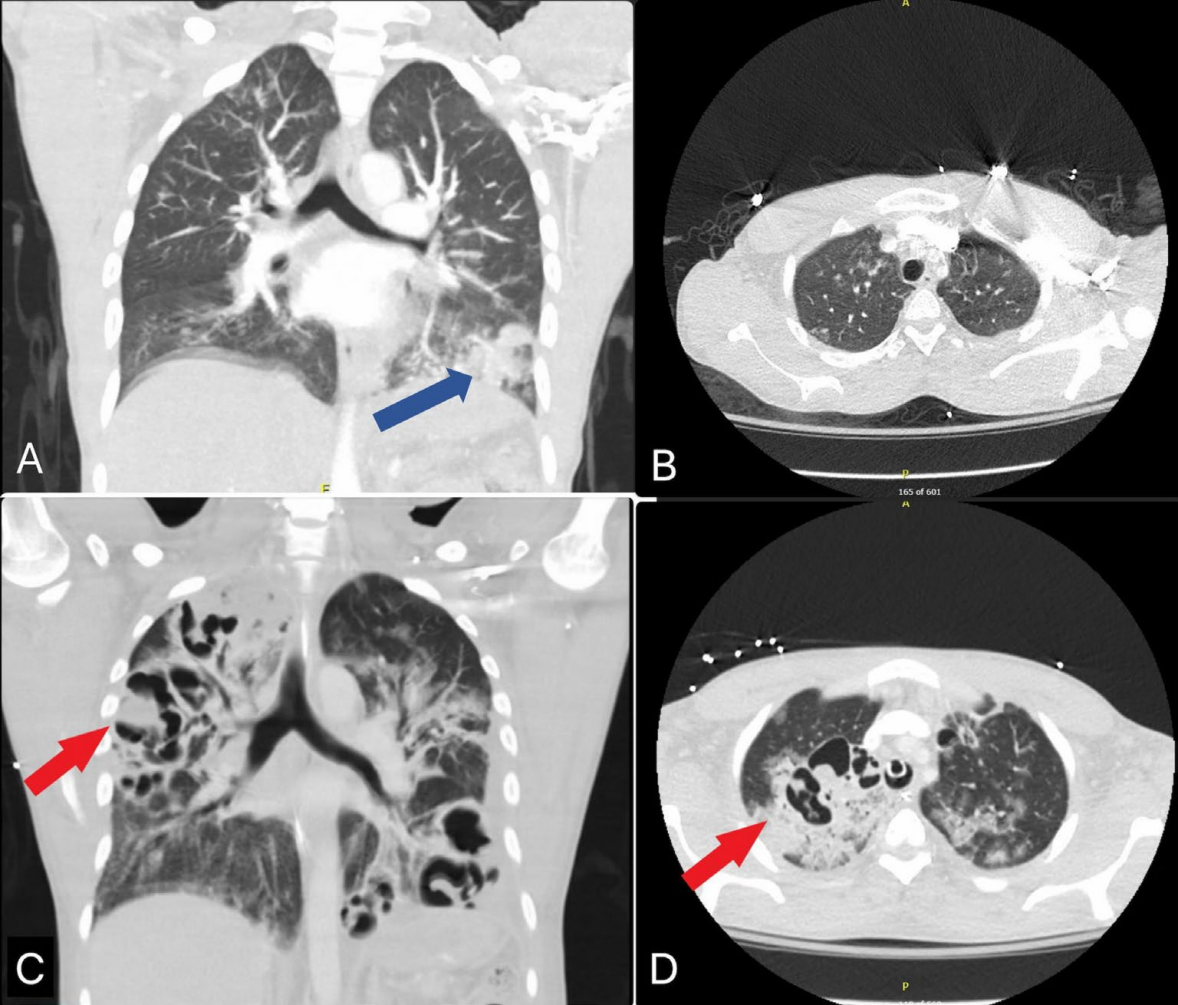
CT imaging of the chest (A) Sagittal view, and (B) Coronal view showing disease process at presentation with large left lower lobe consolidation (Blue arrow); (C) Sagittal view, and (D) Coronal view showing (red arrows) areas of cavitations and multifocal pneumonia.
